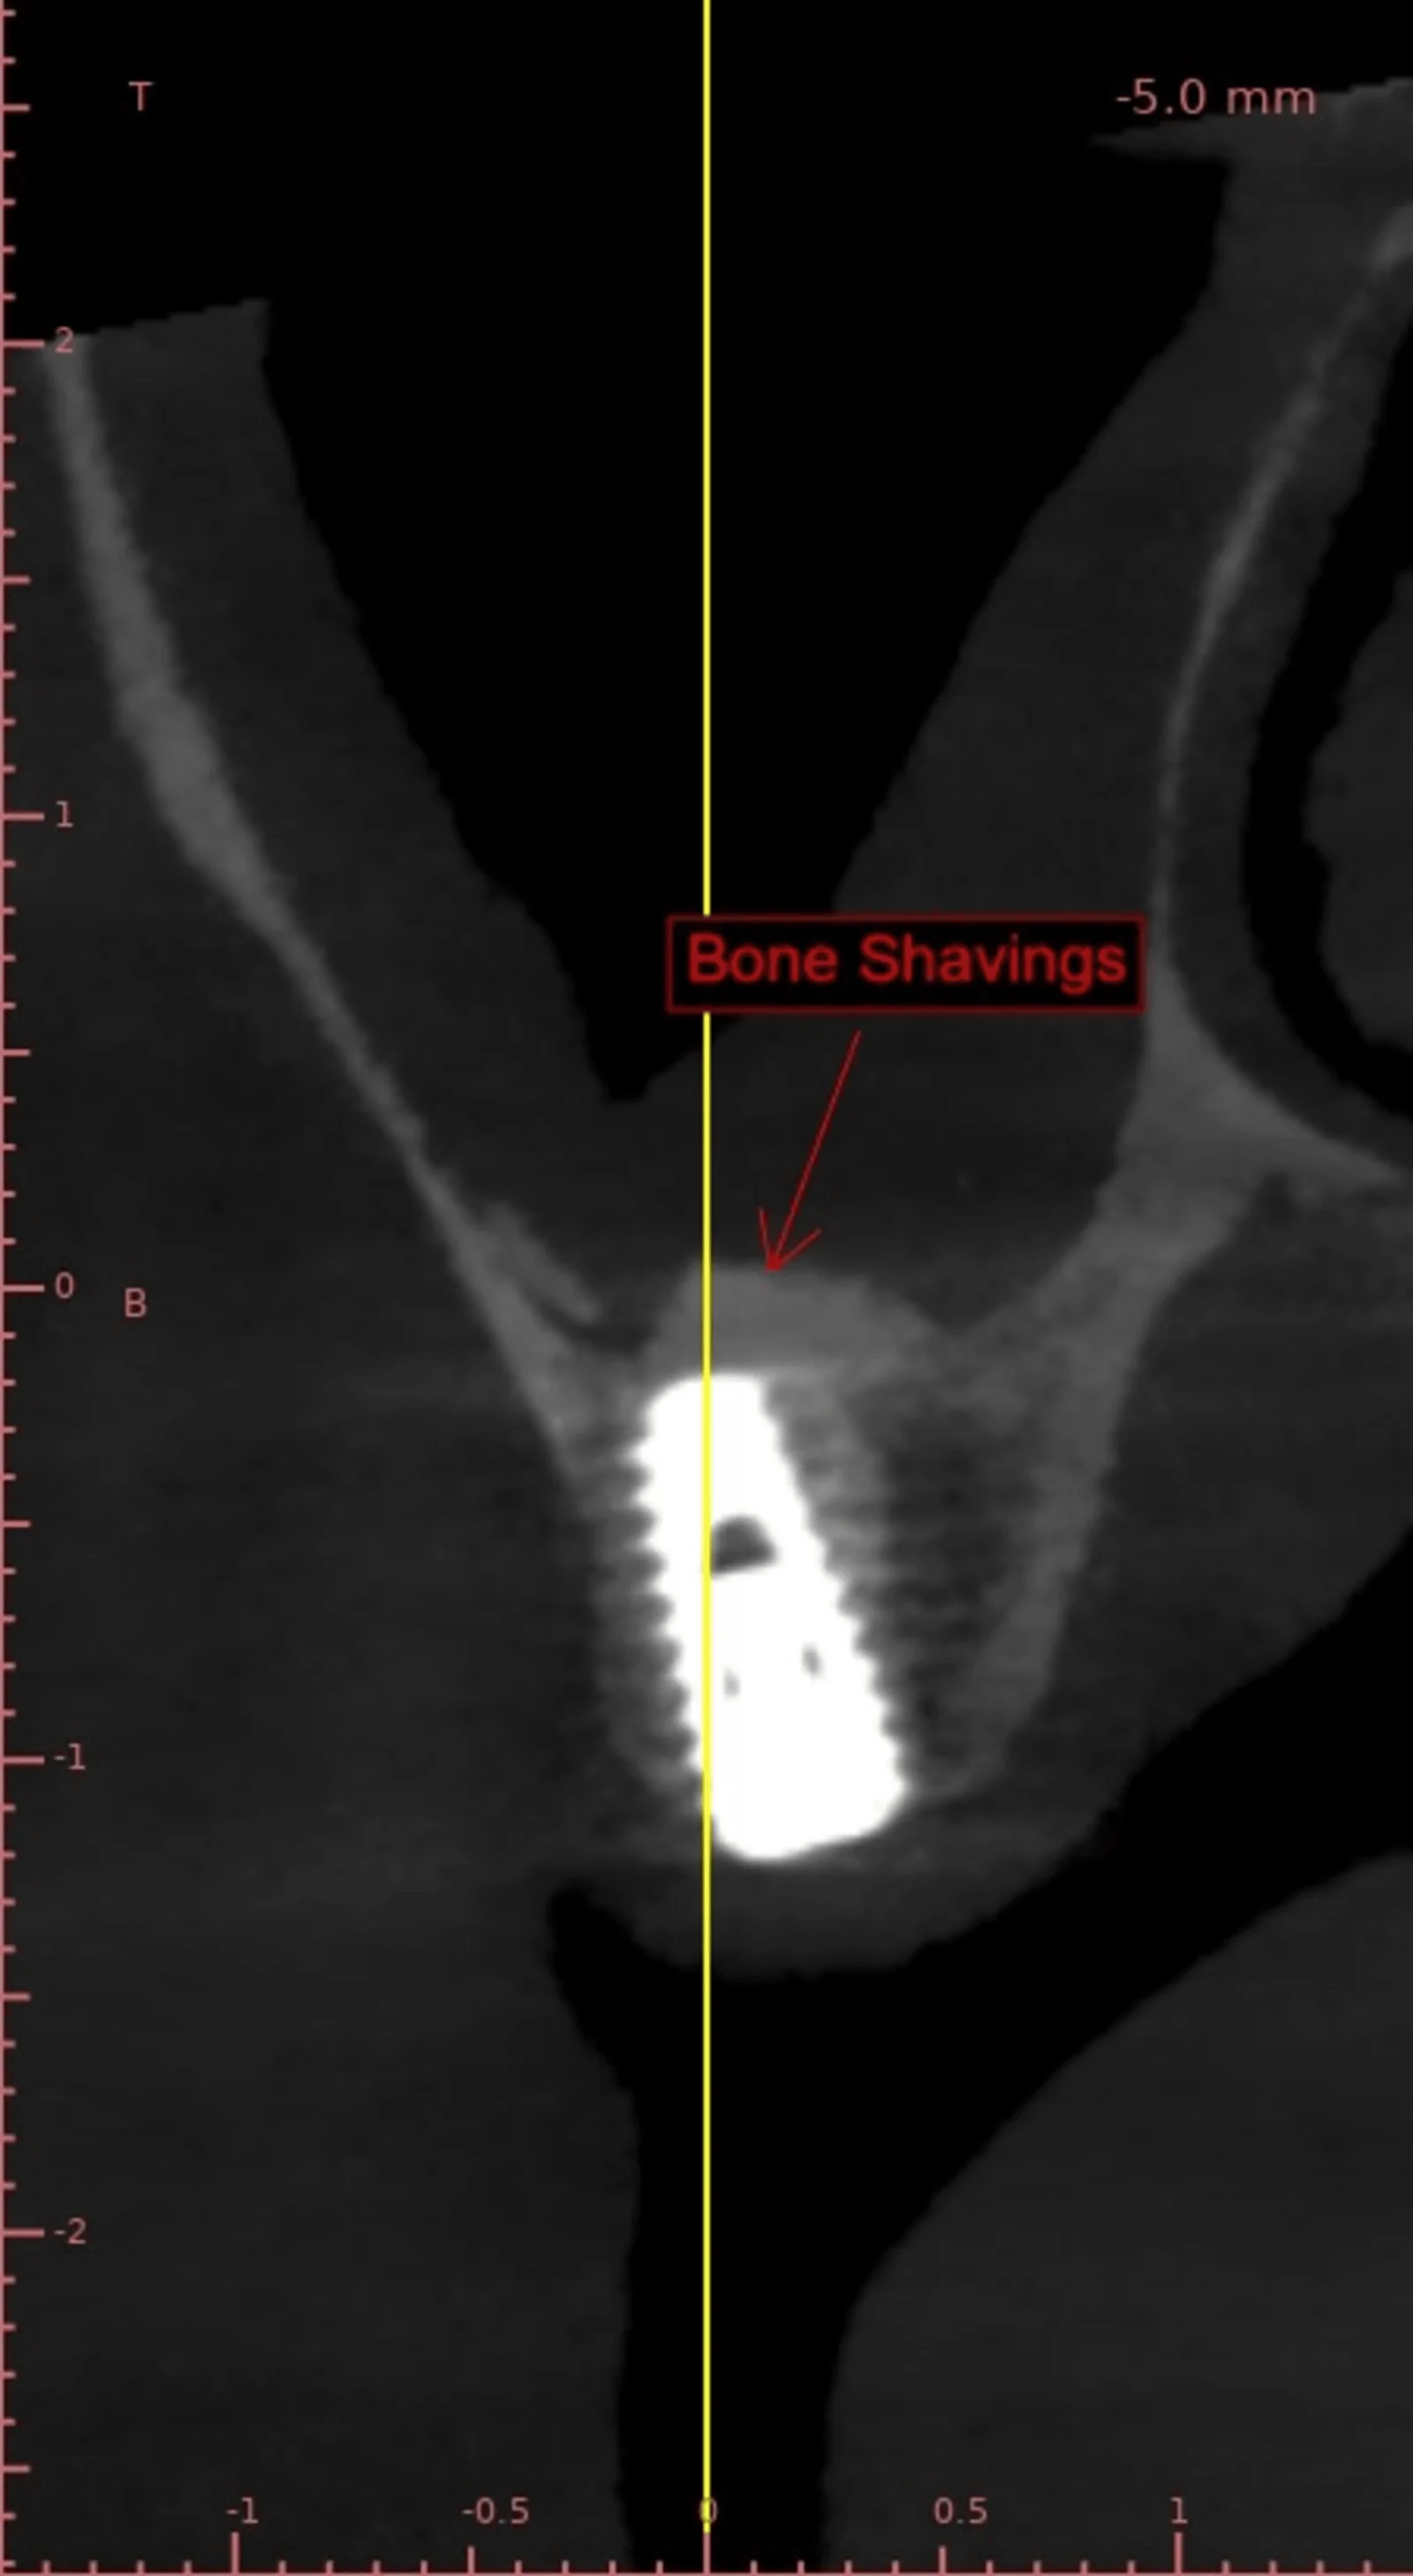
Representative immediate postoperative cone-beam computed tomography image demonstrating compacted autogenous bone chips (arrow) surrounding the implant following osseodensification.
